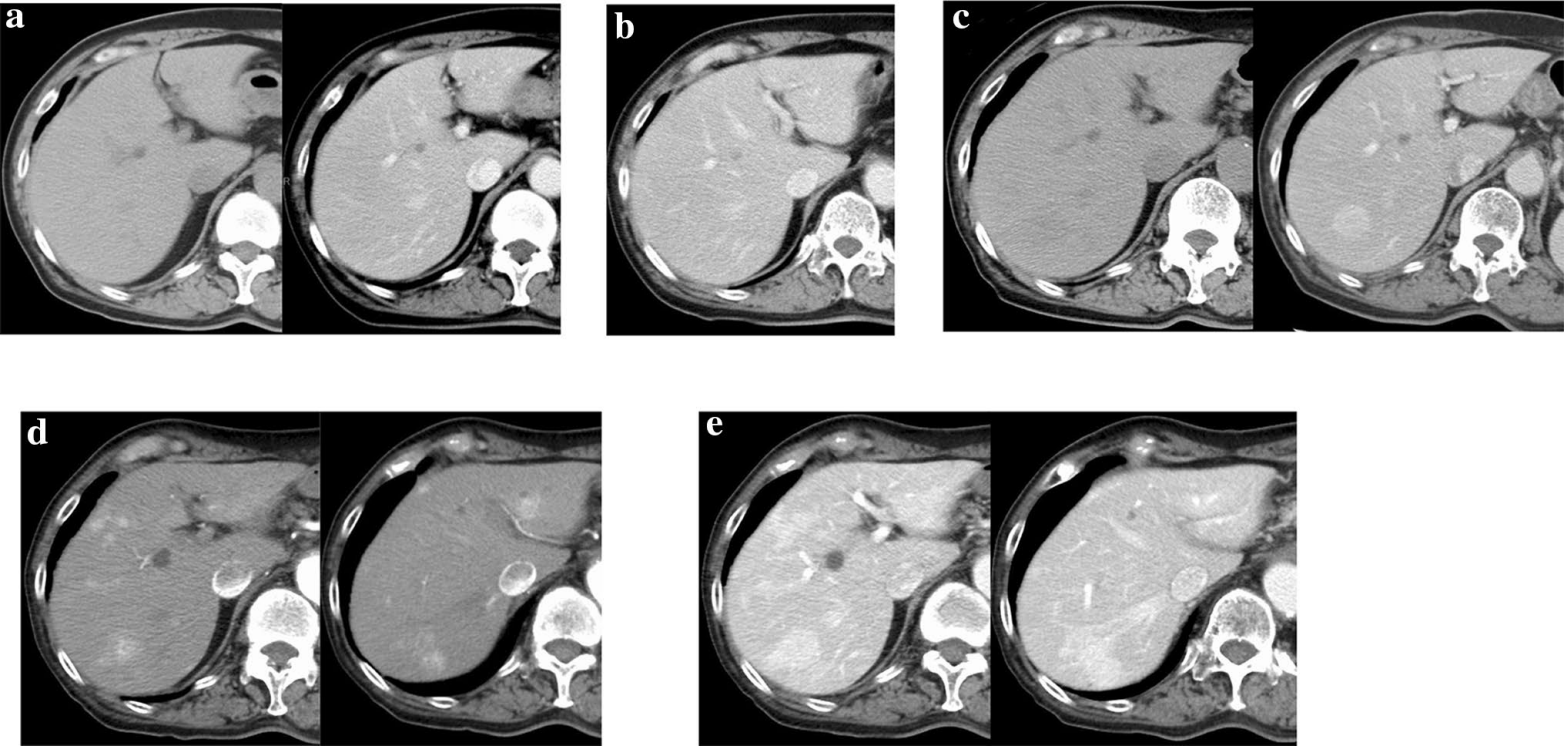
Contrast-enhanced computed tomography. a Plain CT and CECT at 73 years of age showed no mass lesions in the liver. b CECT at 77 years of age shows no mass lesions in the liver. c A 25-mm nodule with hyperdense and clear borders was noted in segment 7 on CECT at 78 years of age. No nodules were detected on plain CT. d The nodules were hyperdense in the arterial phase on CECT at 89 years of age. e The nodules were hyperdense in the portal phase on CECT at 89 years of age. *CECT* contrast-enhanced computed tomography
